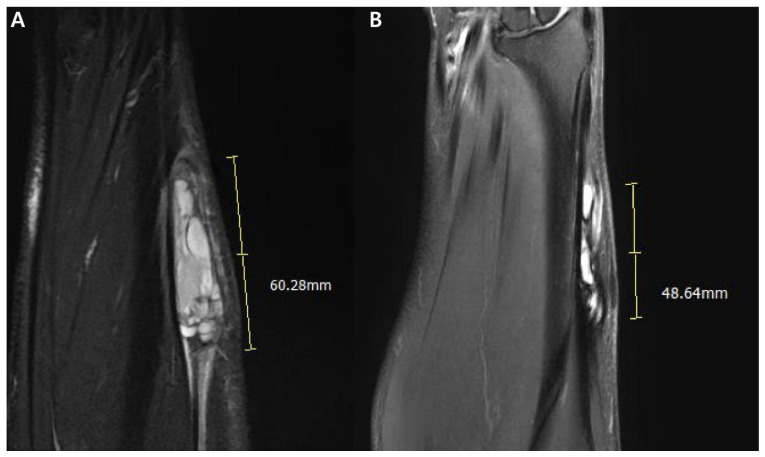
Treatment response after neoadjuvant chemotherapy for synovial sarcoma of 61-year-old-man (monophasic type; same patient with Figure 6): (A) Initial MRI of coronal T2-weighted, fat-suppressed image shows about 6 cm sized multilobulated mass with heterogenous signal intensity of Lt forearm showing ‘bowl of grapes’ sign. (B) After neoadjuvant chemotherapy, follow-up MRI of coronal T2-weighted, fat-suppressed image shows treatment response with decreased size and decrease of peritumoral edema.
